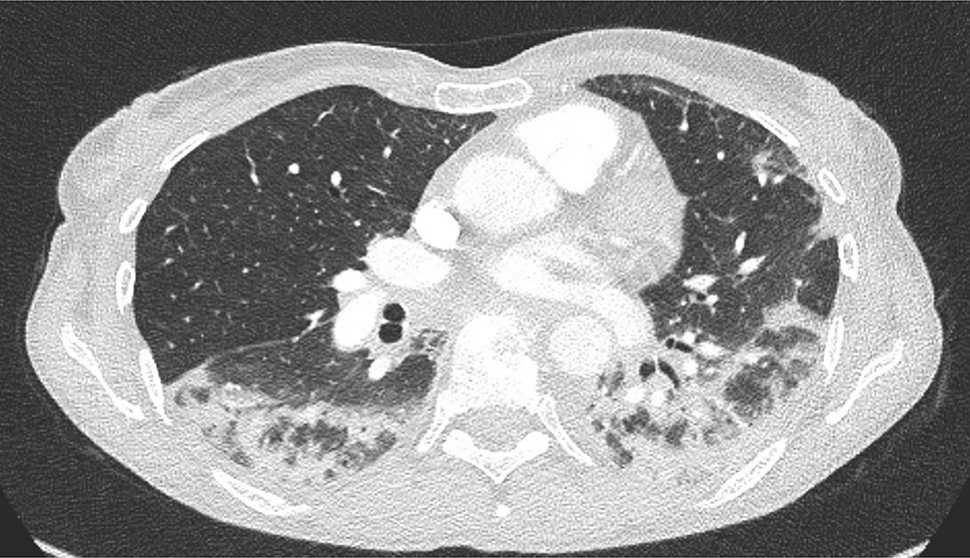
Chest computed tomography scan showing bilateral diffuse pneumonic infiltrates
